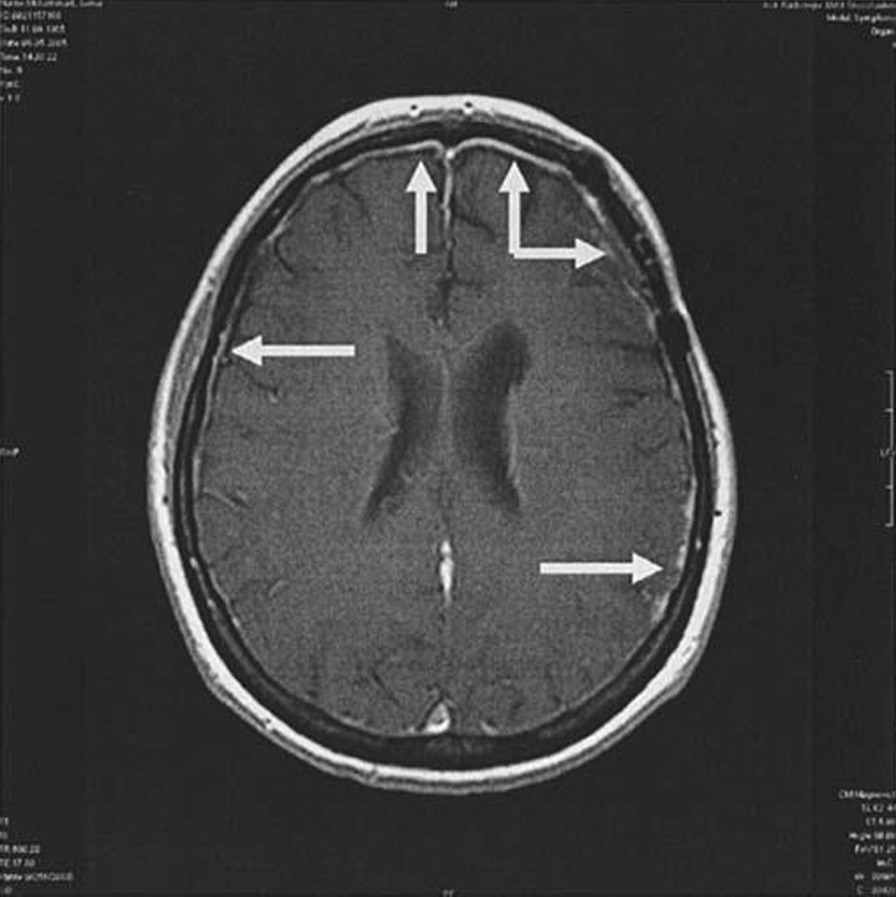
Magnetic resonance imaging (MRI) head - arrows pointing towards meningeal enhancement
